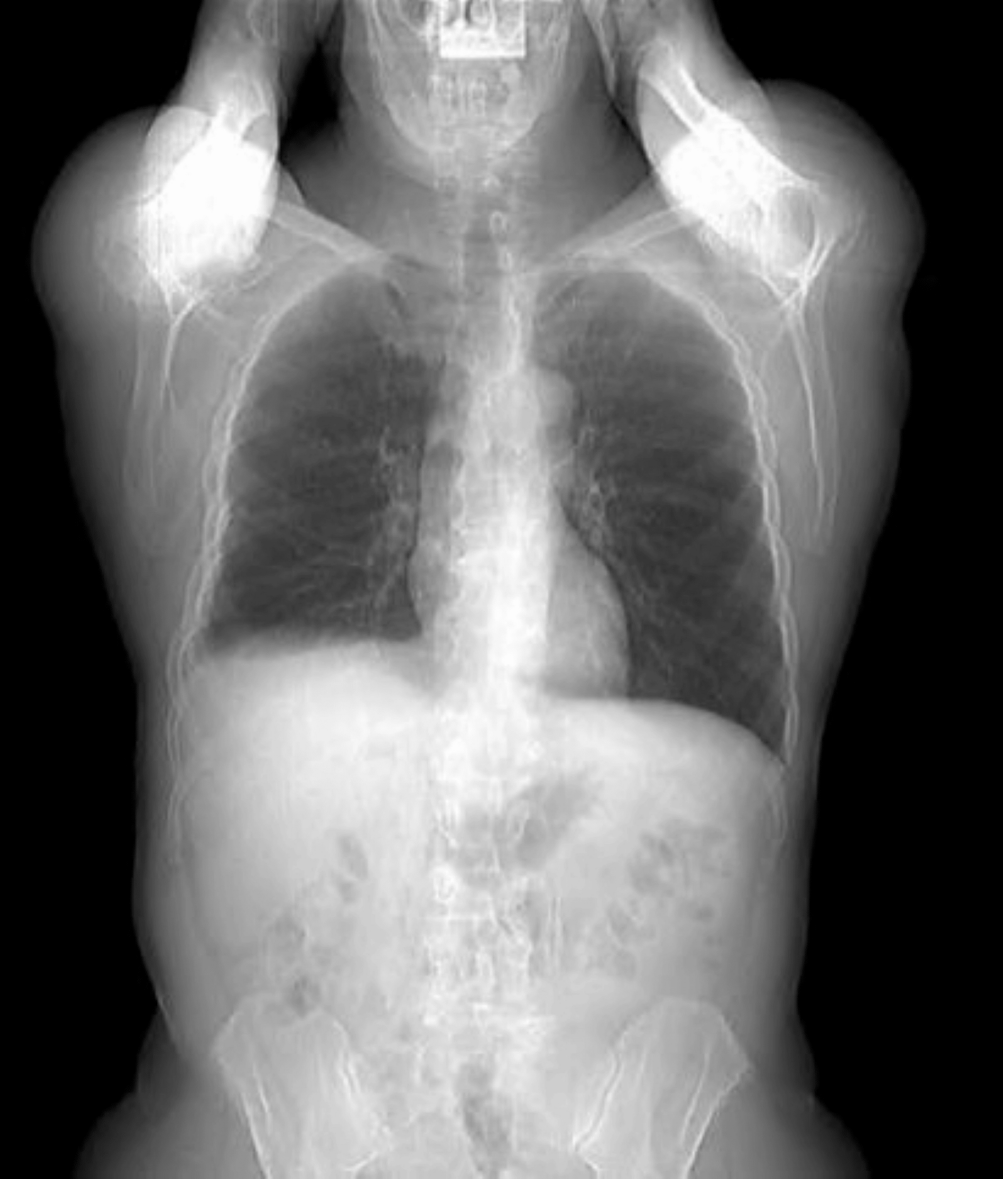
Thoracoabdominal CT scan performed five months after diagnosis (coronal view), with marked improvement after antibiotic therapy.
